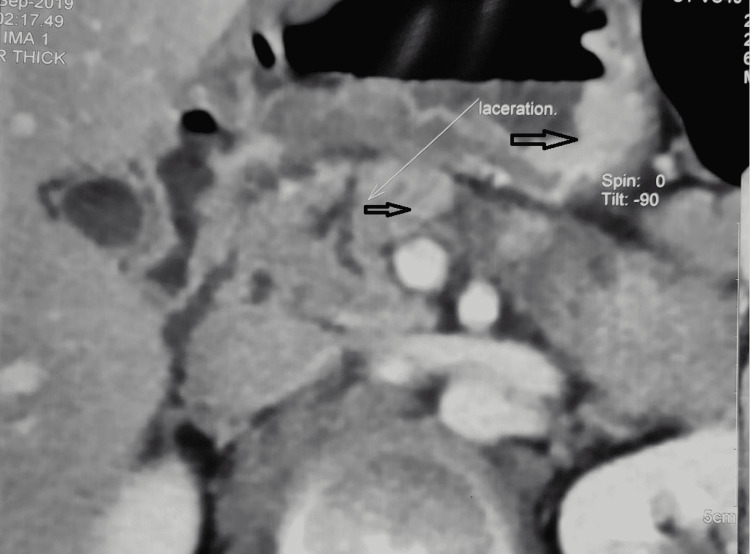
CECT abdomen showing Grade 4 pancreatic injury with lesser sac collection CECT - contrast-enhanced computed tomography; upper black arrow - lesser sac collection; lower black arrow - Grade 4 pancreatic injury
